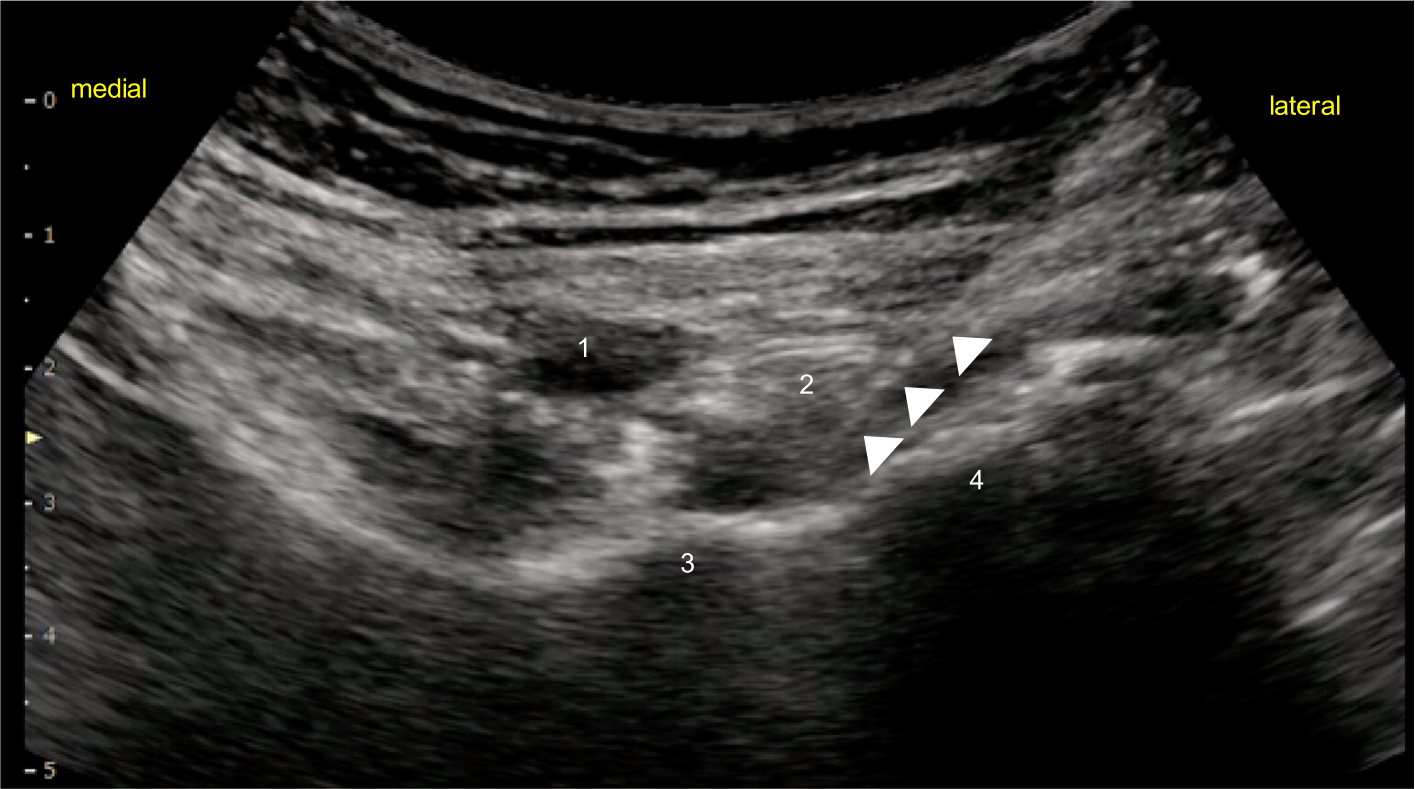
Ultrasound images showing sonoanatomy while performing pericapsular nerve group block. White arrowheads shows the block needle. 1 = femoral artery; 2 = psoas tendon; 3 = iliopubic eminence; 4 = anteroinferior iliac spine
